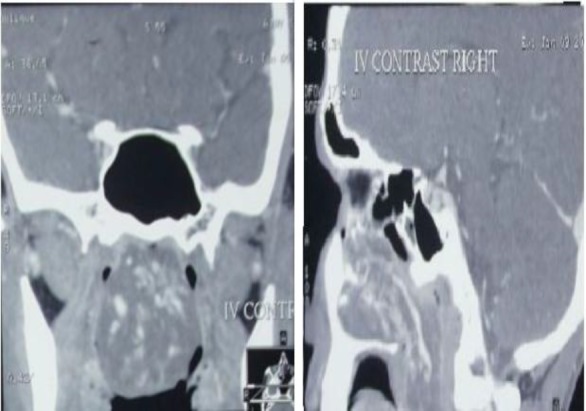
Shows contrast enhanced CT scan (Coronal and Sagittal cut) demonstrating a heterogeneous enhanced mass occluding the whole nasopharynx hanging to the oropharynx
